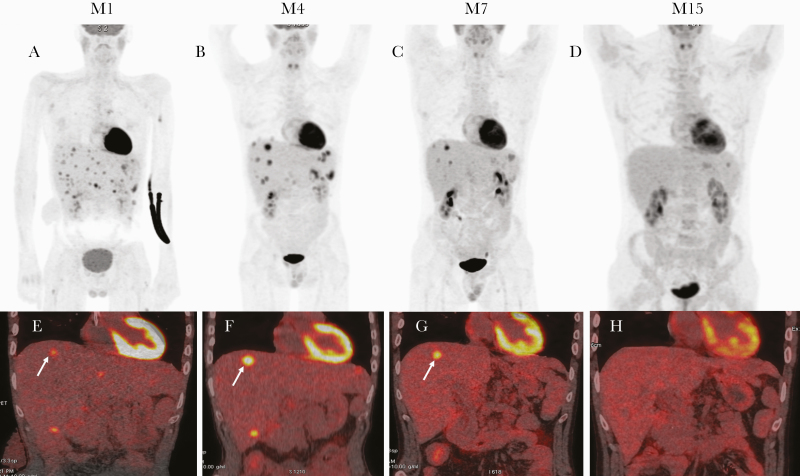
18-FDG positron emission tomography/computed tomography (PET/CT) during disease course. 18-FDG PET/CT scan maximum intensity projection (MIP) showed a metabolic modification with progression of several liver nodules despite the regression in numbers between month 1 (M1) (A) and month 4 (M4) (B), then decreased FDG uptake in the liver abscess and regression in uptake of numbers of lesions at month 7 (M7) (C) and complete regression at month 15 (M15) (D). Fused coronal PET and CT images show several abscesses with a target on the liver dome. In (E) (M1), the arrow indicates a liver node (SUV max, 6; metabolic tumor volume, 2.3 cm^3^). In (F), the arrow indicates an increase in FDG uptake in liver target (SUV max, 11.6; metabolic tumor volume, 4.5 cm^3^) at M4. G, Decreased metabolic activity (SUV max, 9.8; metabolic tumor volume, 2.5 cm^3^) at M7. H, No metabolic activity at M15.
